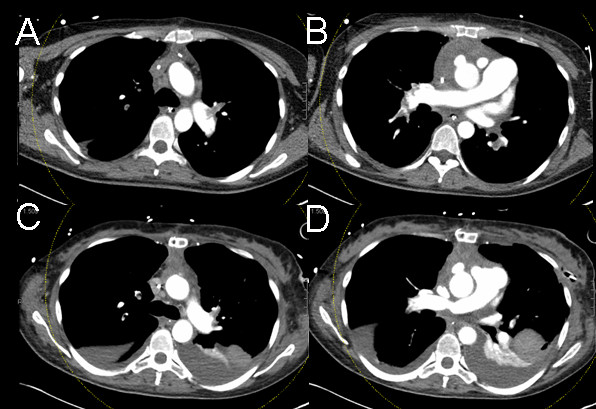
CT-Scan: A & B before surgical embolectomy. C & D directly after surgical embolectomy.
